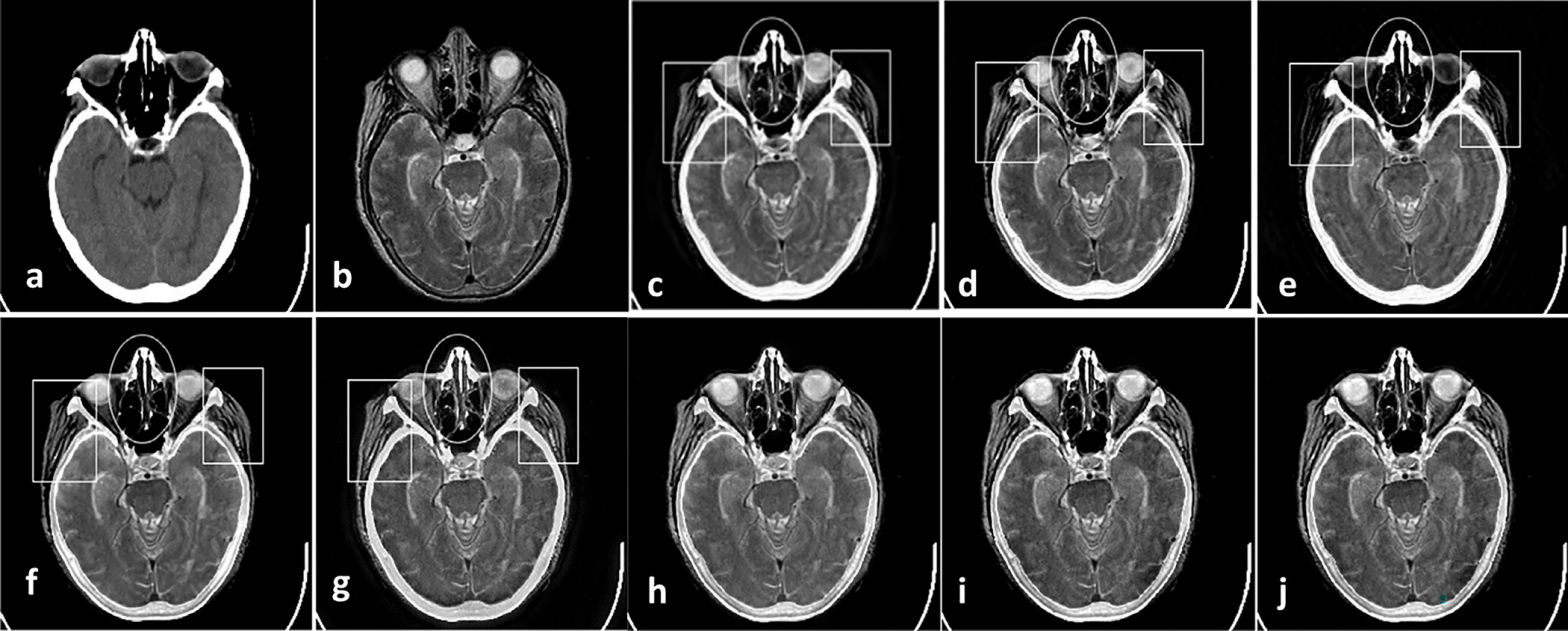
Experiment 6 [53]: Results of fusing CT and MR images of a brain. (a) CT image; (b) MR image; (c) NNSST [93]; (d) FMSAP [95]; (e) CST [97]; (f) ST-NSST [96]; (g) MFDF-NSST [53]; (h) the proposed method with PCA, (i) max, and (j) weighted averaging model, respectively.
